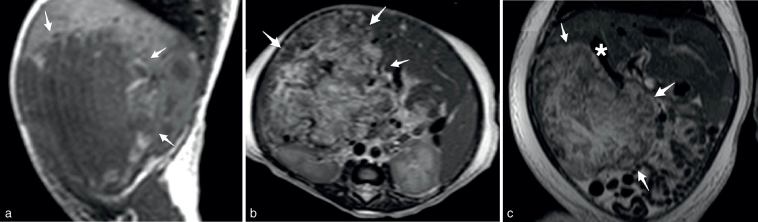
MRI showed a heterogeneous hepatic mass (arrows) in the right lobe, mostly hypointense on *T*
